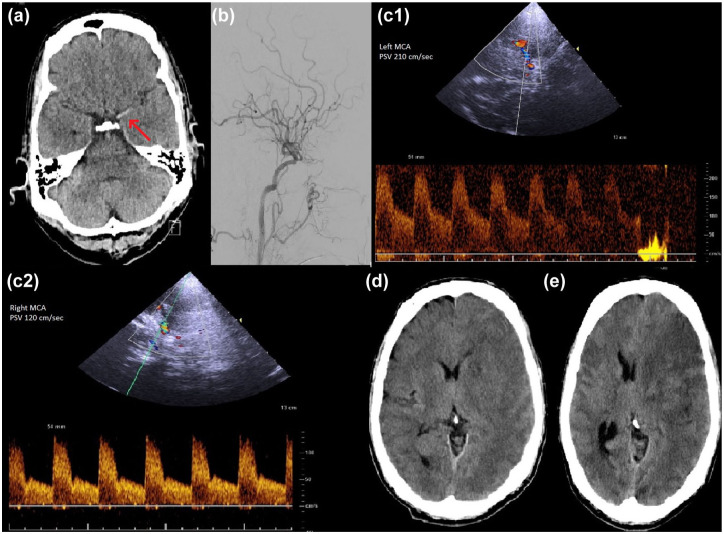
Exemplary case of a patient with right hemiparesis and aphasia (NIHSS score 19 points). (a) Initial CT scan showing hyperdense media sign (red arrow), proximal occlusion of the left MCA. (b) Digital subtraction angiography after successful mechanical recanalization of the left MCA (TICI 2c). (c1) Transcranial Duplex sonography of the left MCA with increased PSV (210 cm/s) 5 h after recanalization compared to (c2) the right MCA. (d, e) CT scans showing malignant swelling of MCA infarction with midline shift 6 and 16 h after successful recanalization. MCA, middle cerebral artery; NIHSS, National Institutes of Health Stroke Scale; PSV, peak systolic velocity; TICI, thrombolysis in cerebral infarction.
